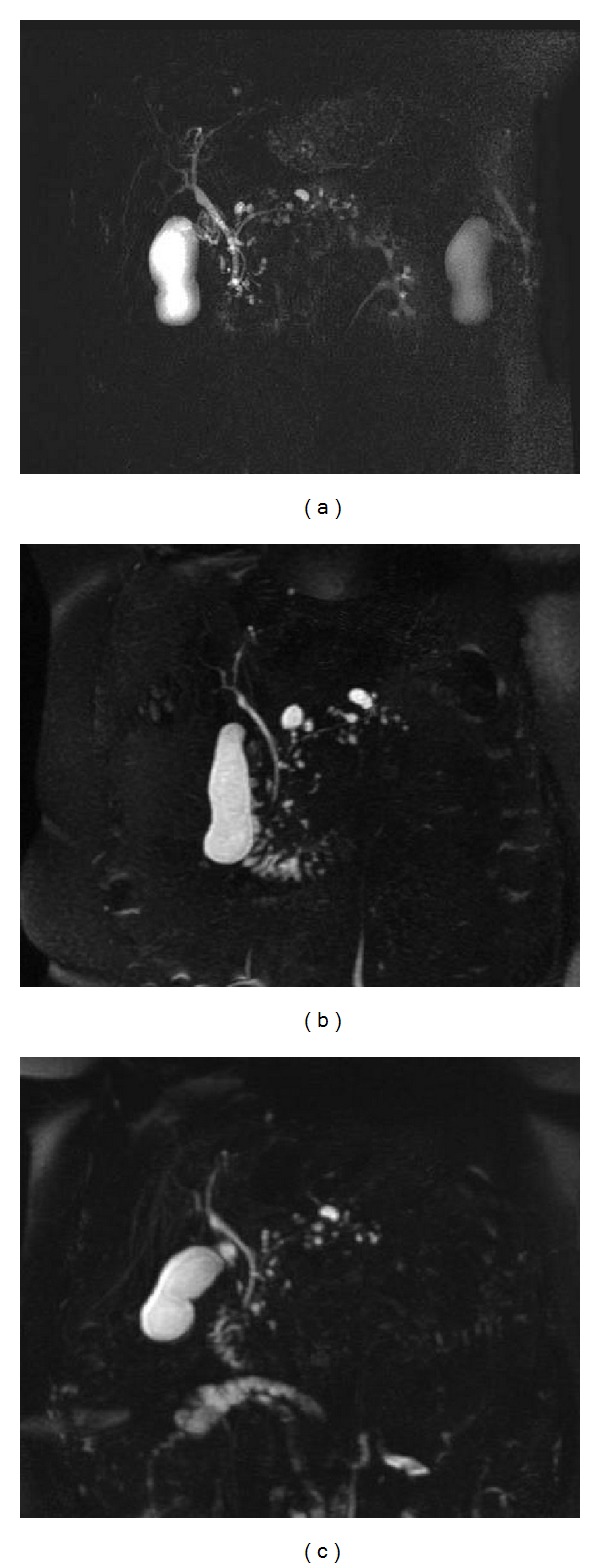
Coronal MRCP acquisitions in an asymptomatic 70-year-old female patient with an incidental radiological finding of multiple pancreatic cystic lesions; MRCP exams were performed in 2009 (a), in 2012 (b), and in 2013 (c). Multiple small cystic lesions in the pancreatic parenchyma are clearly depicted in the three MRCP acquisitions, some of them showing a typical connection to the main pancreatic duct. This typical radiological pattern suggests the diagnosis of multifocal BD-IPMNs. No main pancreatic duct dilatation is observed. Cystic lesions do not show intraluminal solid components or mural nodules. Over time the MRI monitoring initially showed a mild enlargement of the lesions (from a to b) and then a size-reduction (from b to c). As reported in literature, IPMNs in a multifocal setting could also be managed in a safe and reliable mode.
